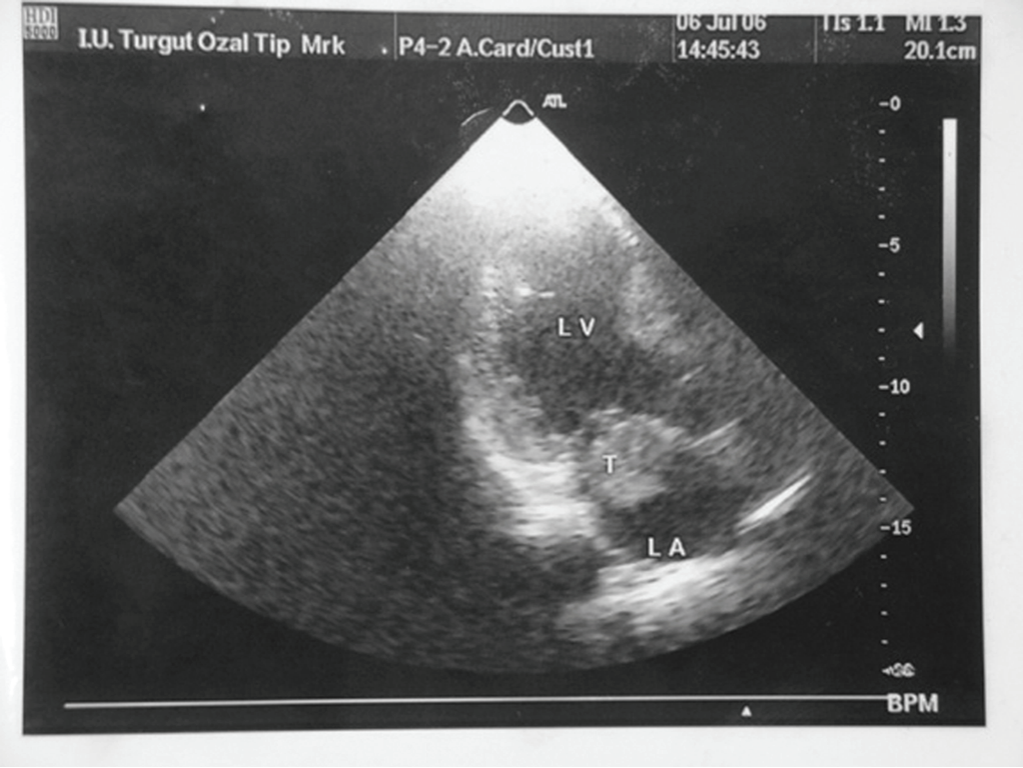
Transesophageal echocardiographic visualization of the thrombus
